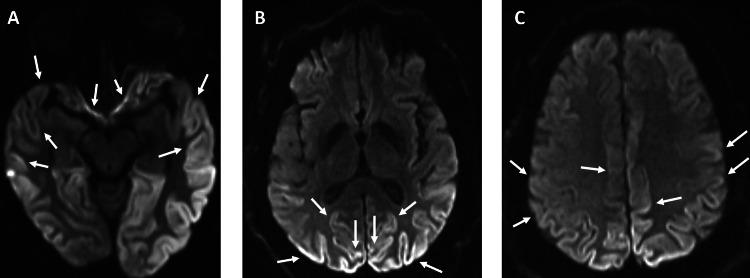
MRI (axial view) of Case 1 with cortical hyperintensity in DWI sequence The arrows point to the cortical ribboning of the bilateral (A) temporal, (B) occipital, and (C) parietal lobes. DWI: diffusion-weighted imaging
